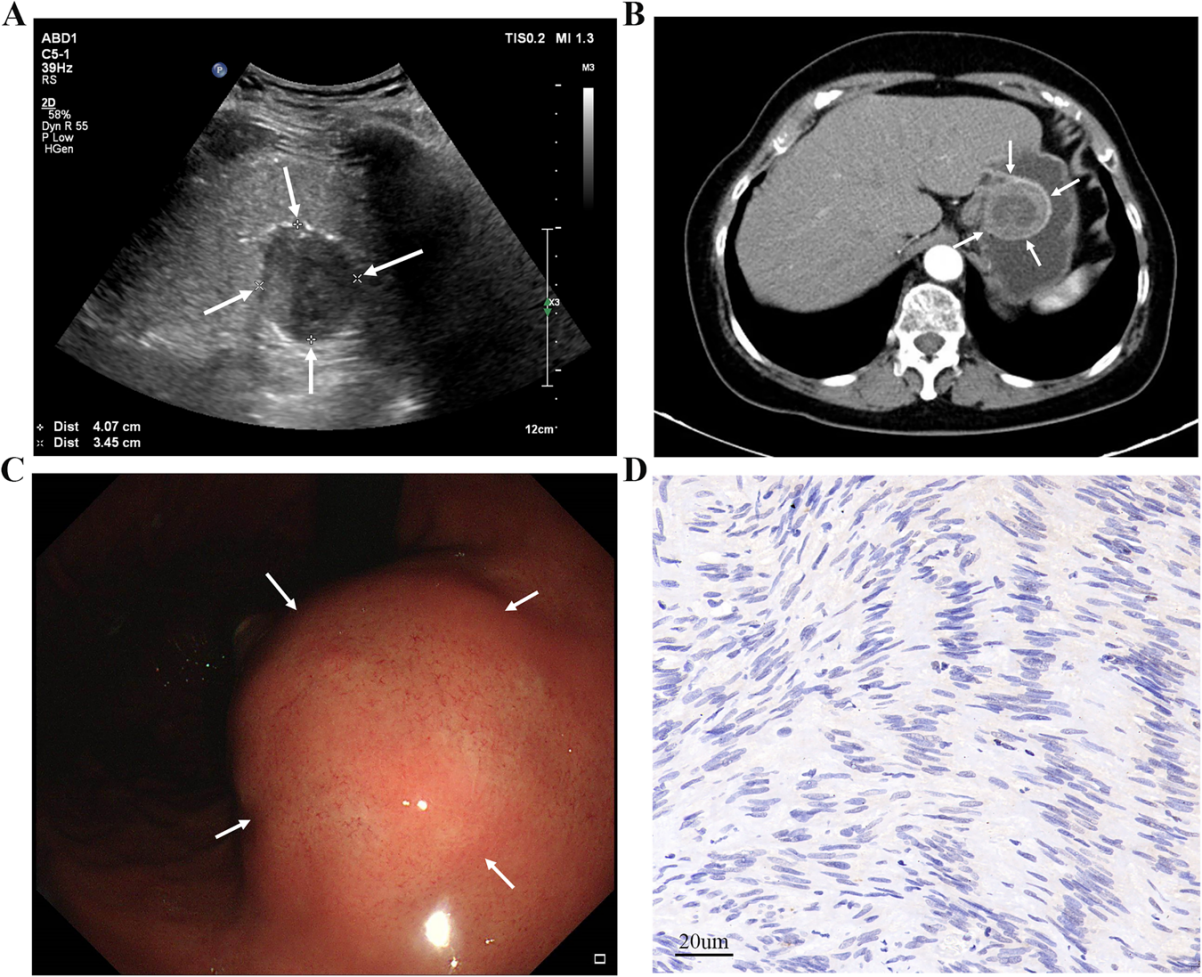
A An approx 75-year-old woman underwent gastric oral contrast ultrasonography, revealing a heterogeneous low echo under the gastric fundus mucosa, which was round and about 4.1 × 3.5 cm. The shape was regular with a smooth surface, and the boundary was clear. The hierarchical structure of the gastric wall was clear and not obviously thickened. B Submucosal round cystic solid mass at the fundus of the stomach, with clear boundaries and convex shape toward the gastric cavity, about 4.3 × 4.0 cm, presented enhanced scan with mild uneven enhancement on enhanced computed tomography. C Electronic gastroscopy found a 4.0 × 5.0 cm submucosal bulge in the fundus of the stomach. The surface was smooth, and the color was the same as the surroundings. D The pathological result showed that the gastrointestinal stromal tumor was 4.6 × 4.5 × 3.5 cm (scale bar: 20 μm)
